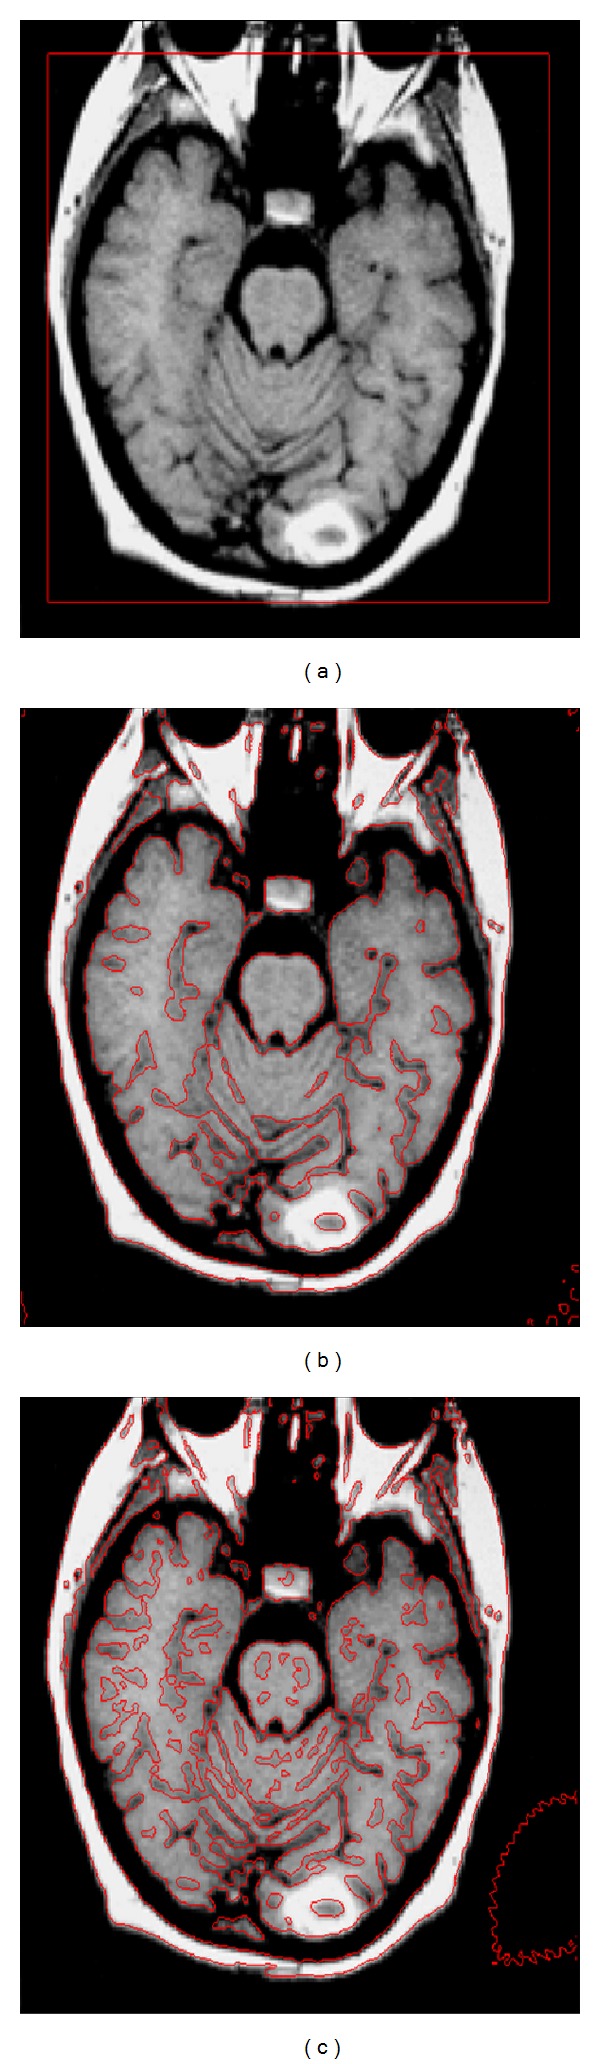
2D brain MR image segmentation using different values of σ_2_. (a) Initial contour; (b) final contour with σ_1_ = 3.0 and σ_2_ = 1.0; (c) final contour with σ_1_ = 3.0 and σ_2_ = 0.5.
